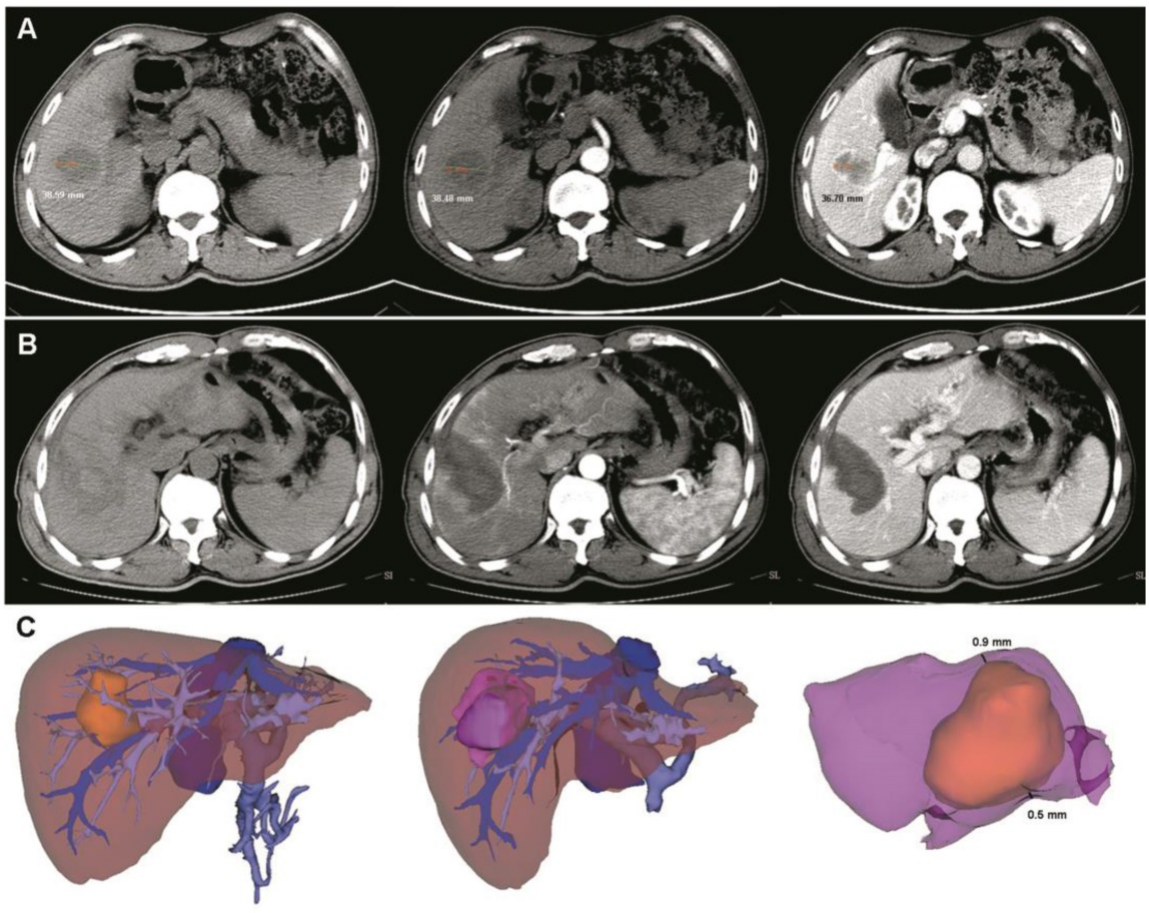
Pre- and post- operative 3D-CT reconstruction and image fusion. (A) Pre-operative two-dimension computed tomography (plain scan, arterial and venous phases). The tumor size was about 38.59 mm. (B) After ultrasound-guided RFA combined with 3D-CT reconstruction, a low-density area was observed (plain scan, arterial and venous phases). (C) Pre- and post-operative 3D-CT reconstruction and image fusion. The yellow mass indicates the tumor and the purple mass indicates the ablation area. After image fusion, the safety margin was measured in a multidimensional approach. In this case, the thinnest safety margin was 0.6 mm. RFA, radiofrequency ablation.
